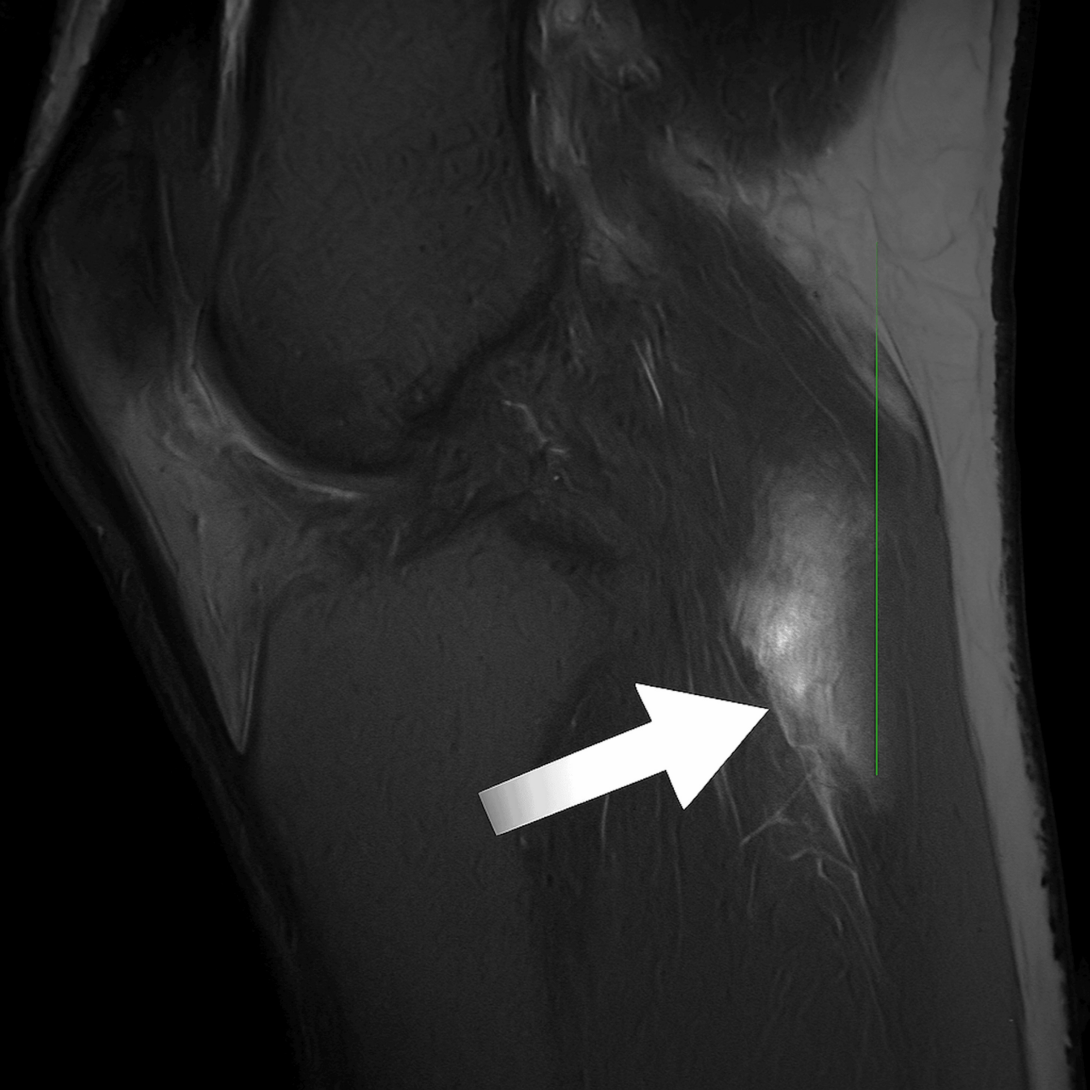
MRI results showed a 5.2 x 1.3 x 6.6 cm soft tissue fluid collection just deep to the skin along the posterolateral left knee (indicated with the arrow). MRI, magnetic resonance imaging.
